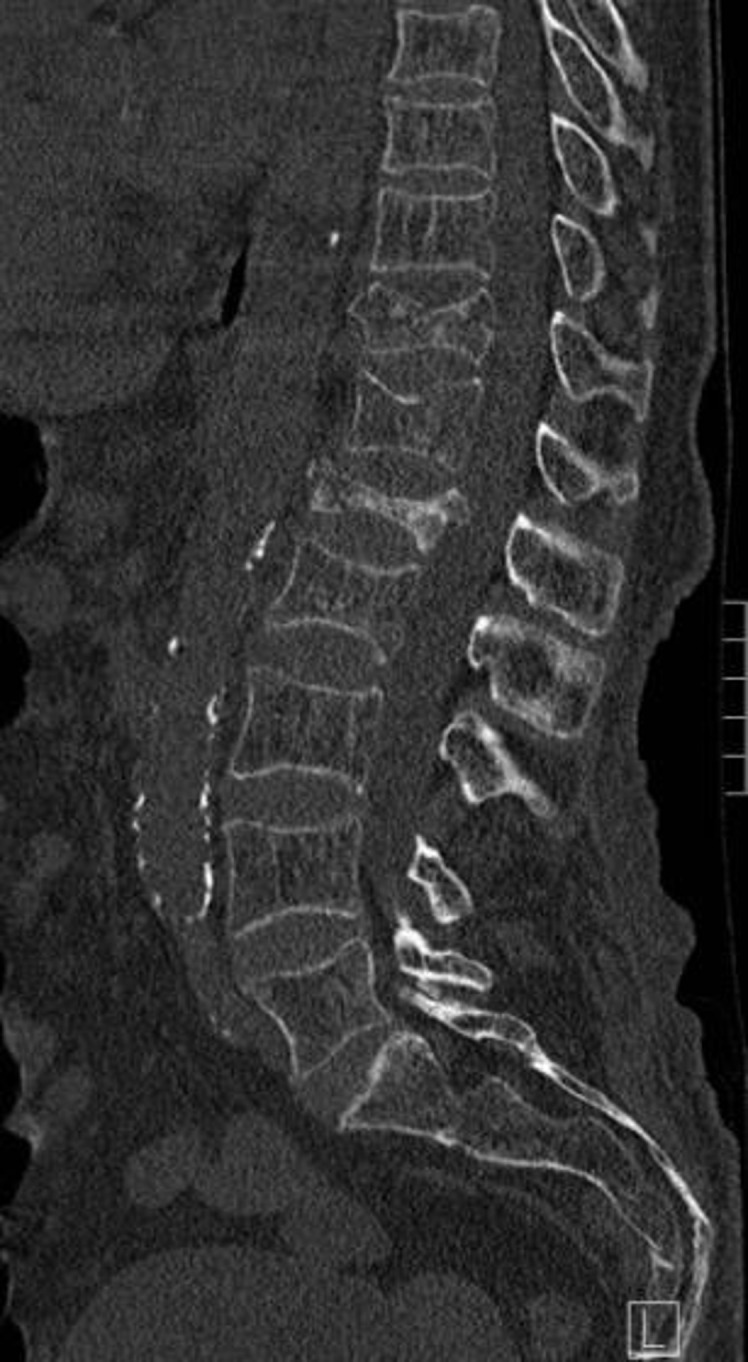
Sagittal reformatted CT of the lumbar spine in an 83-year-old female demonstrating severe osteoporotic compression fracture of L1, moderate compression fracture of T11 and mild compression fracture of L2
